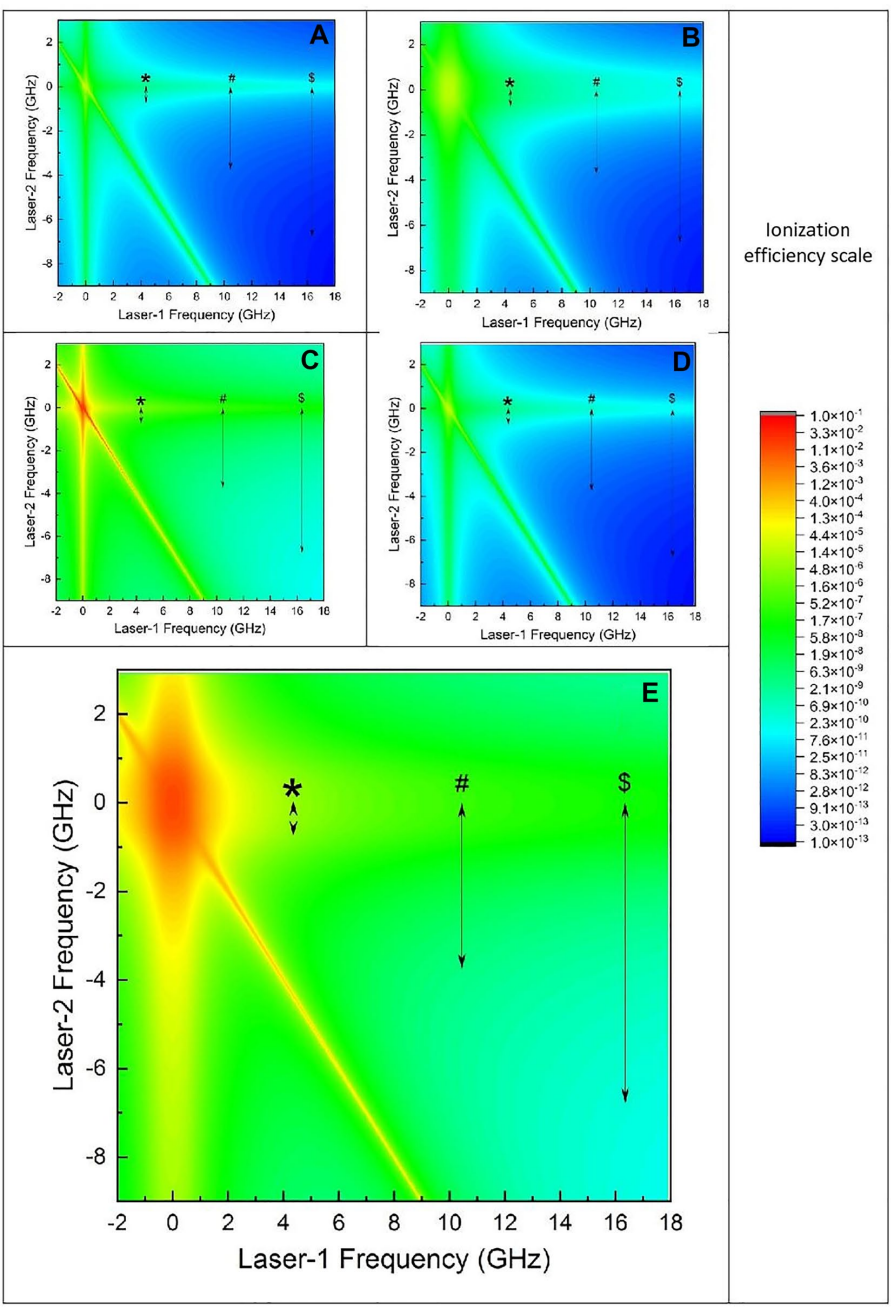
Two dimensional contour of ionization efficiency of even isotope of Radium. The frequency scale is referenced to the resonance frequency of the even isotope. The resonance position of $$F = \frac{3}{2} \to F^{^{\prime}} = \frac{5}{2} \to F^{^{\prime\prime}} = \frac{5}{2}$$ hyperfine transition of ^223^Ra for the second excitation transition with reference to ^224^Ra (*), ^226^Ra (#) and ^228^Ra ($) are also marked. (A) Bandwidth of both excitation lasers is 0 MHz; Peak power density of the excitation and ionization lasers are 10 W/cm^2^, 10 W/cm^2^ and 10 W/cm^2^ respectively; Full angle divergence of the atomic beam is 0° (i.e., No Doppler broadening) (B) Bandwidth of both excitation lasers is 500 MHz; Peak power density of the excitation and ionization lasers are 10 W/cm^2^, 10 W/cm^2^ and 10 W/cm^2^ respectively; Full angle divergence of the atomic beam is 0° (i.e., No Doppler broadening) (C) Bandwidth of both excitation lasers is 0 MHz; Peak power density of the excitation and ionization lasers are 10 W/cm^2^, 10 W/cm^2^ and 10,000 W/cm^2^ respectively; Full angle divergence of the atomic beam is 0°. (i.e., No Doppler broadening) (D) Bandwidth of both excitation lasers is 0 MHz; Peak power density of the excitation and ionization lasers are 10 W/cm^2^, 10 W/cm^2^ and 10 W/cm^2^ respectively; Full angle divergence of the atomic beam is 30° (E) Bandwidth of both excitation lasers is 500 MHz; Peak power density of the excitation and ionization lasers are 10 W/cm^2^, 10 W/cm^2^ and 10,000 W/cm^2^ respectively; Full angle divergence of the atomic beam is 30°.
